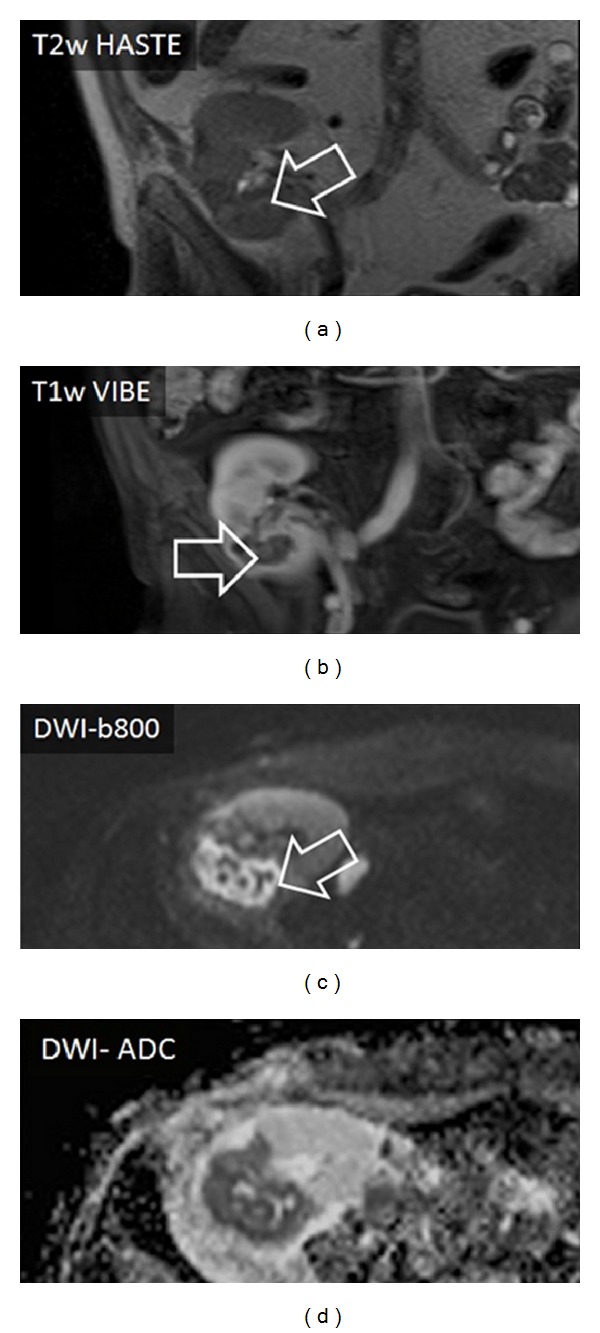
Sixty-five-year-old male patient with an abscess in the kidney transplant (right pelvic region). The abscess formation could be easily detected with DWI-b800 and correlating DWI-ADC present distinctive signal alterations. T2w imaging showed only marginal alterations whereas T1w imaging with contrast defined the abscess superiorly (arrow).
